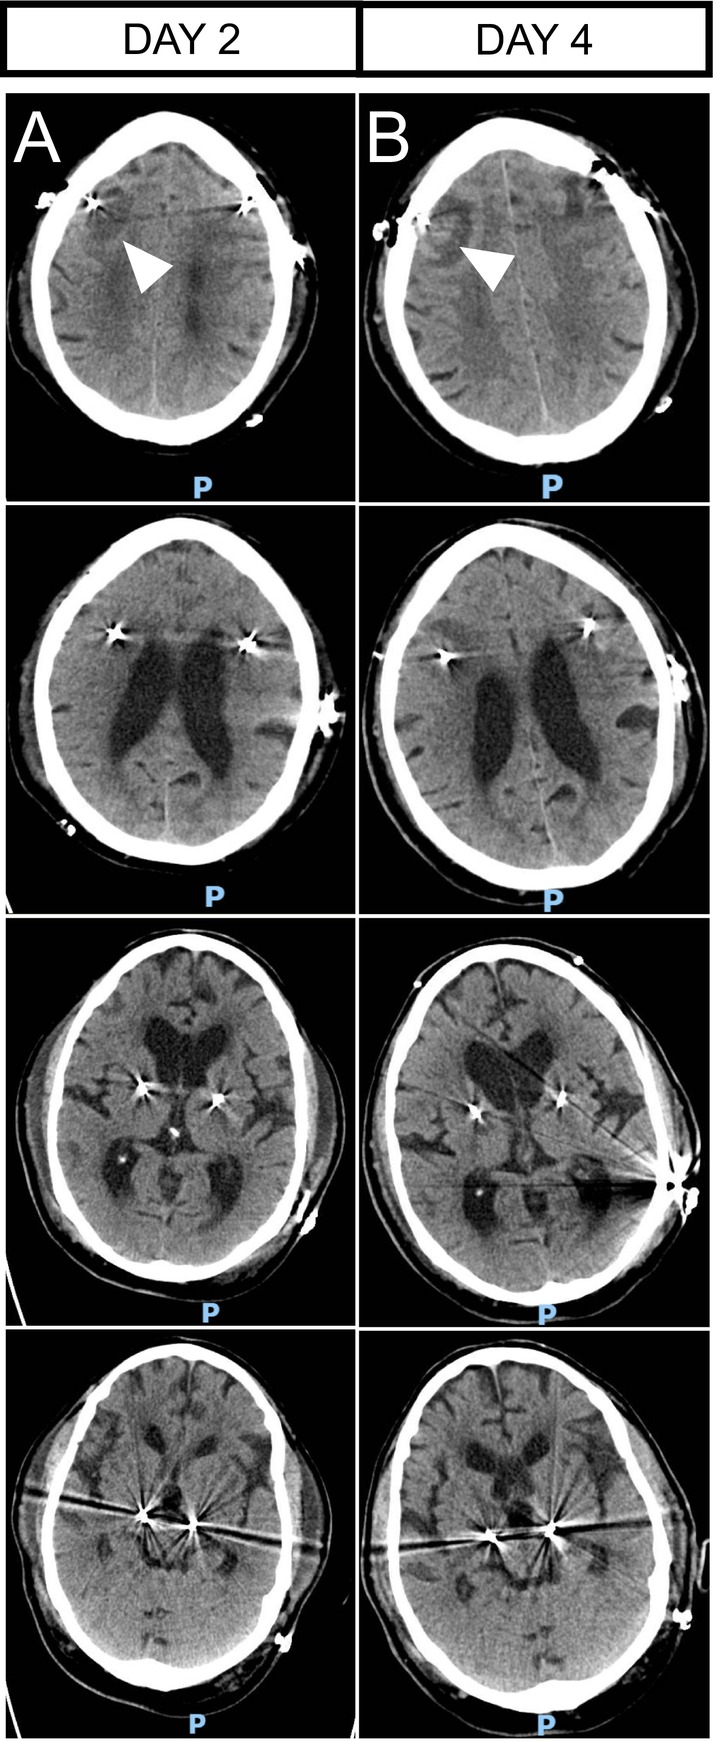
Exemplary radiological findings of longitudinal CT scans. (A) CT on postoperative day two showed bifrontal hypodensities around the electrodes. (B) CT on postoperative day 4 revealed progressive edema (marked with arrows).
